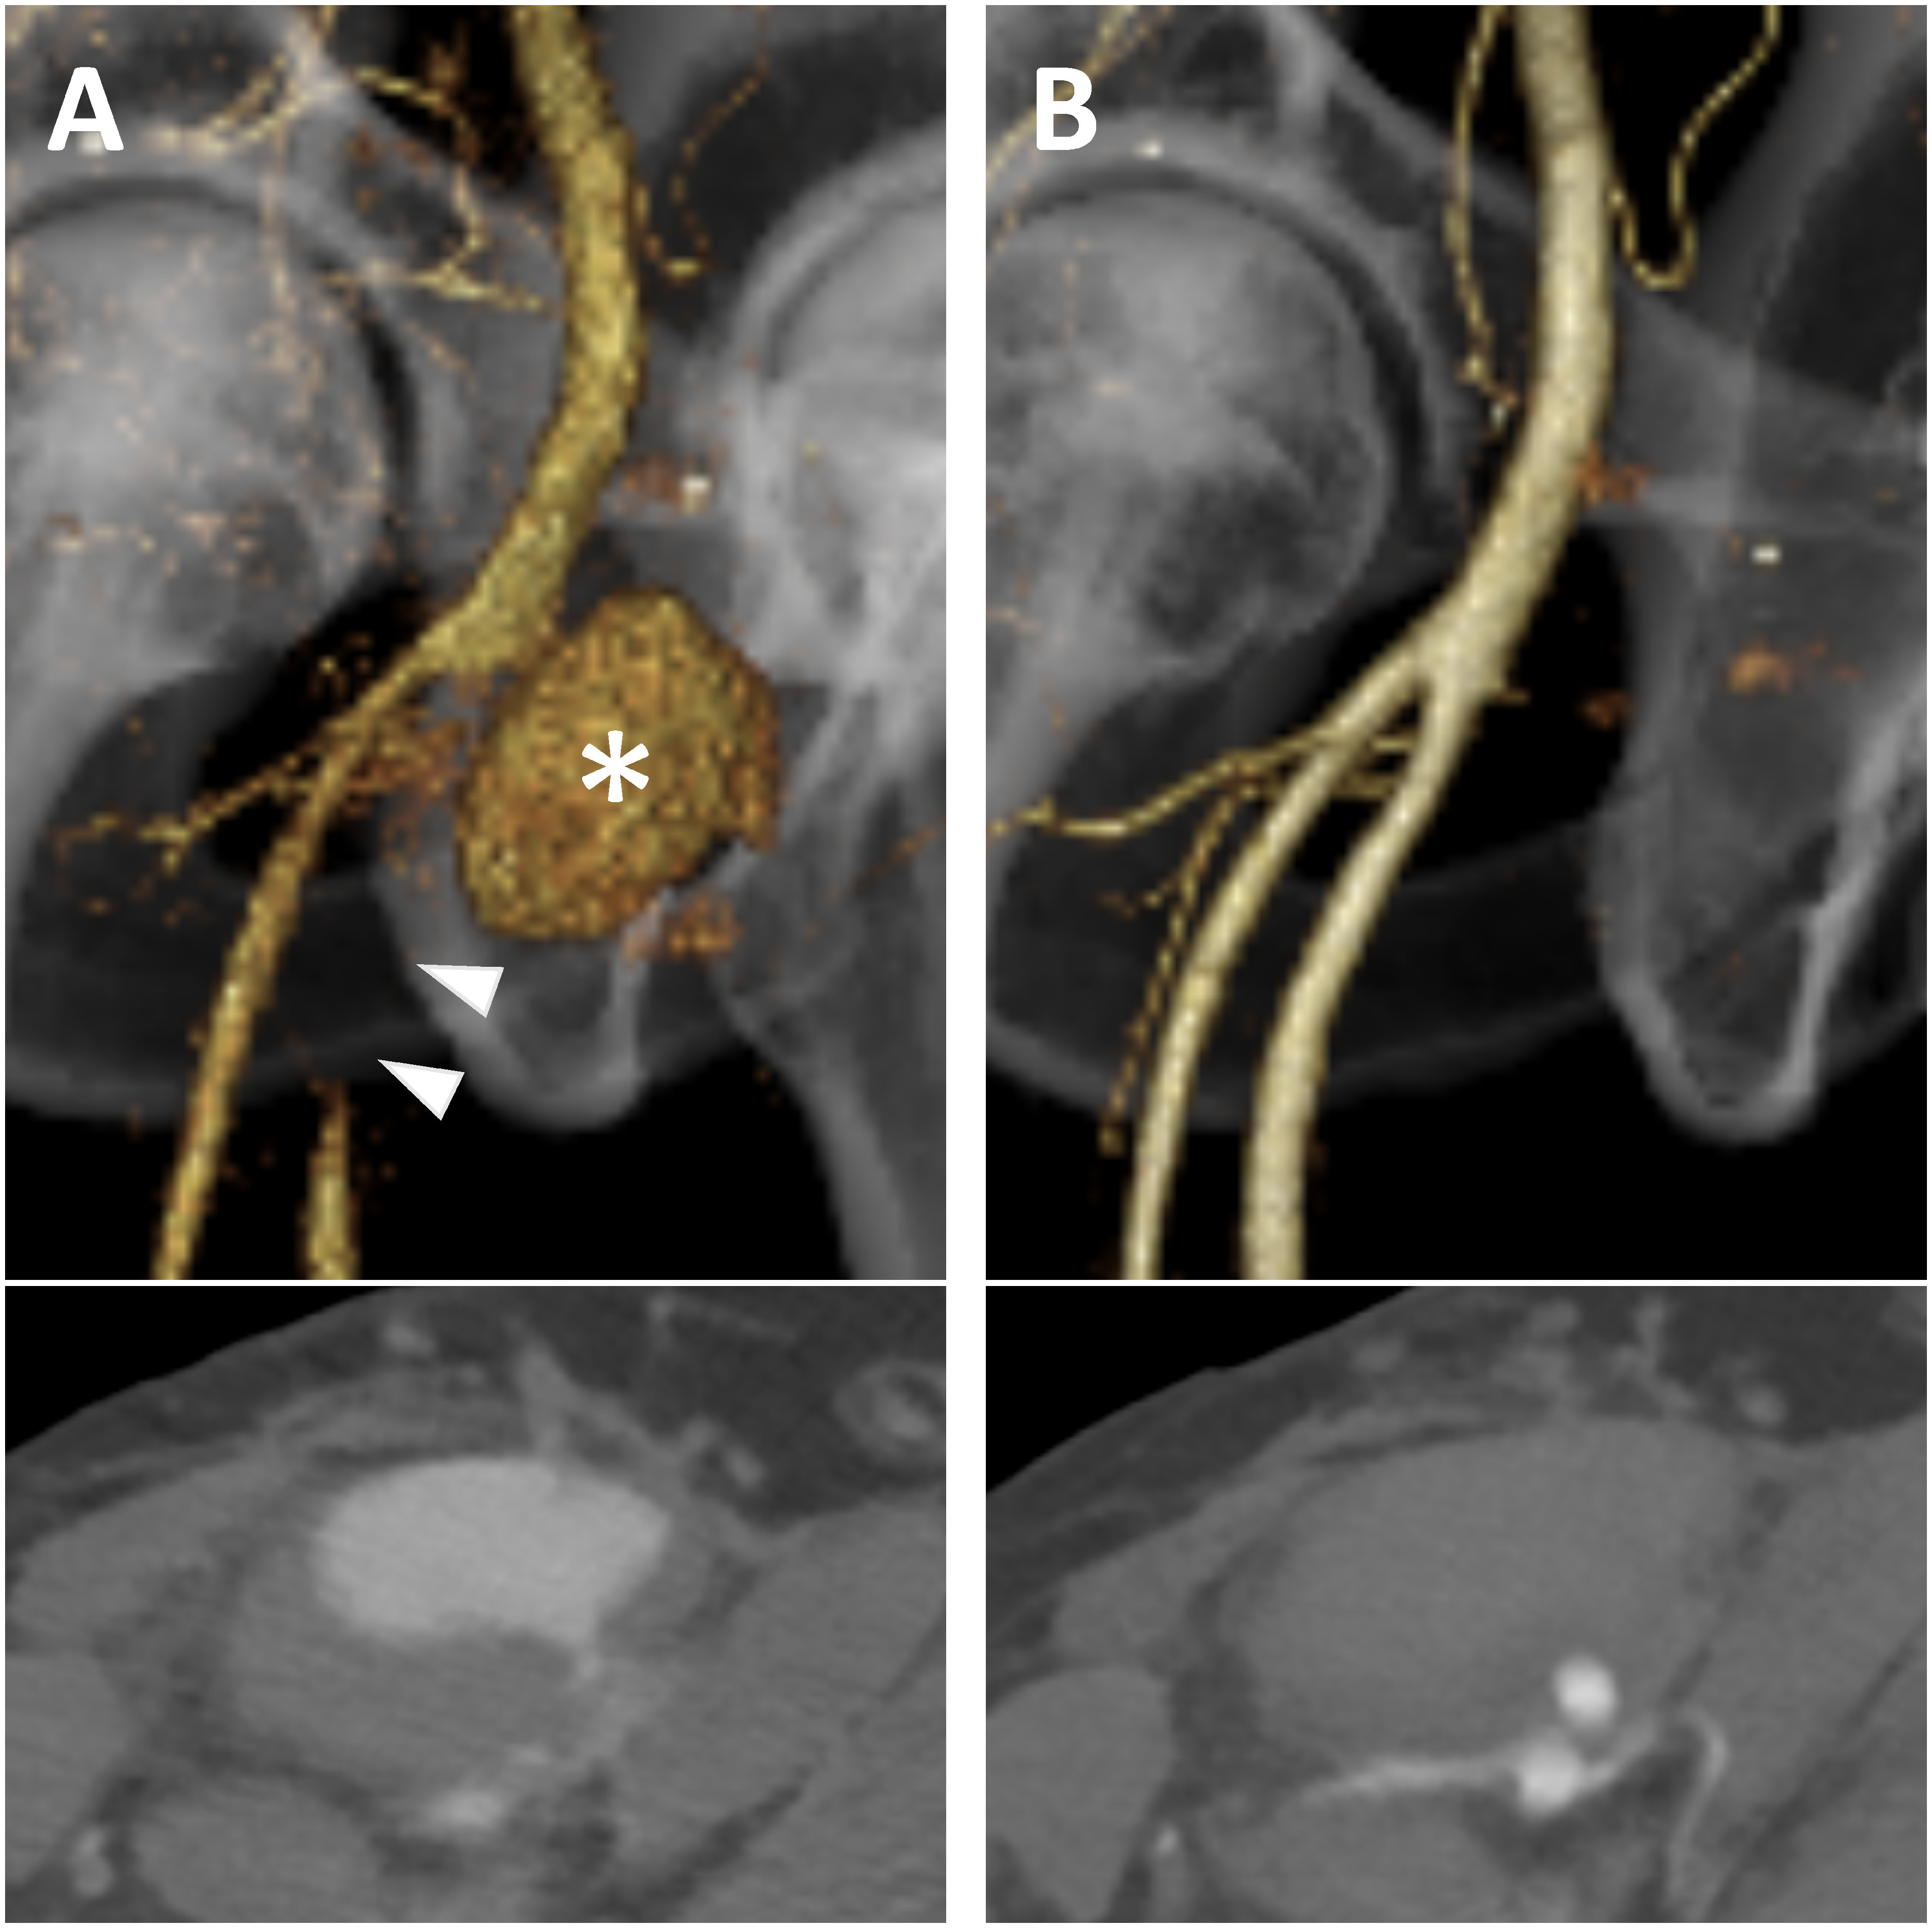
Fig. 1 (A) Digitally recreated three-dimensional (3D) image of computed tomography angiography shows the pseudoaneurysm of the common femoral artery (asterisk), complicated with severe stenosis of the superficial femoral artery due to compression by the pseudoaneurysm (arrowheads). (B) This is a 3D image 5 days after the ultrasound-guided thrombin injection, showing disappearance of the pseudoaneurysm and resolution of the external compression to the superficial femoral artery.
